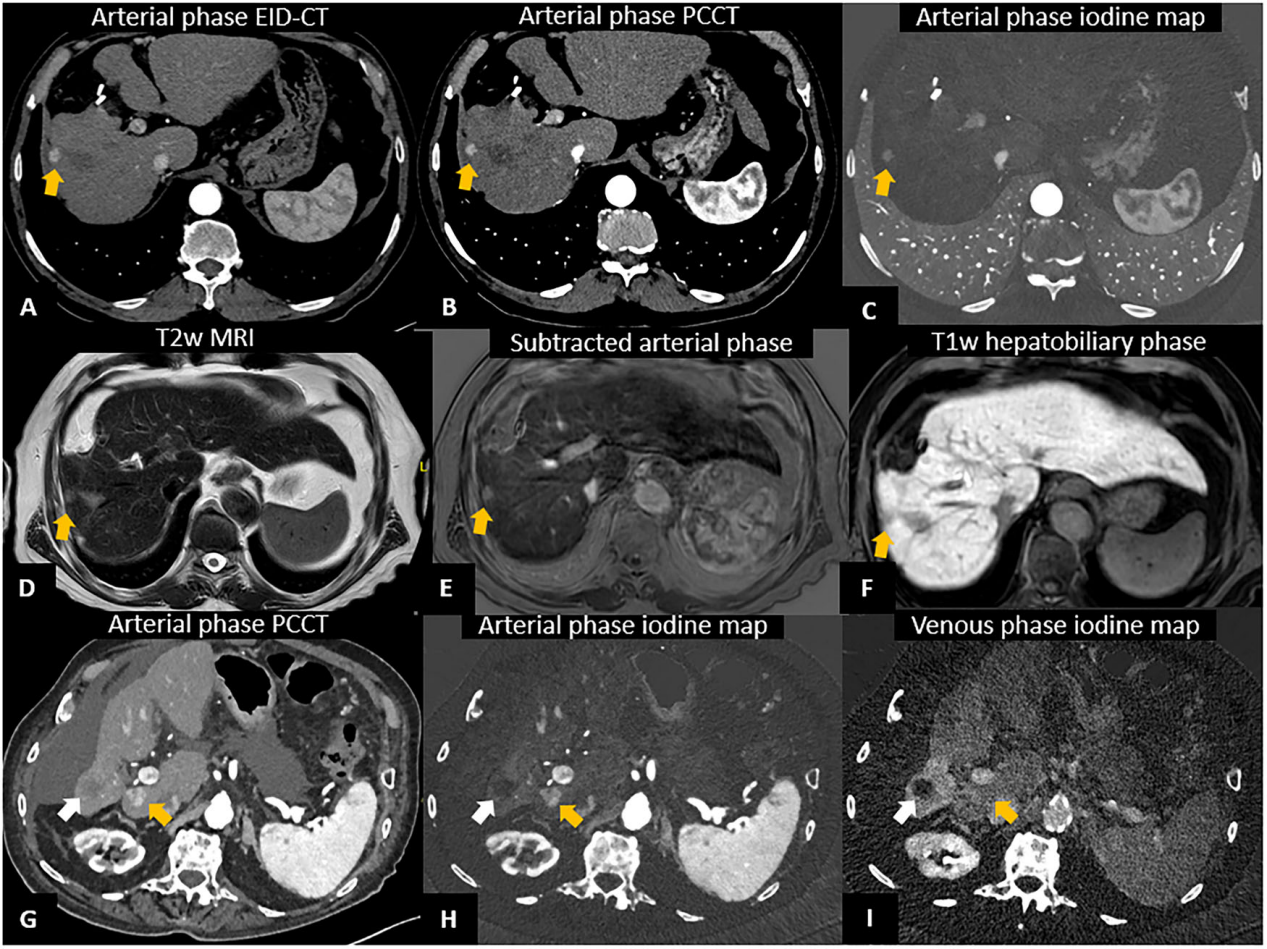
PCCT in hepatocellular carcinoma (HCC). A 75-year-old man with a previous resection for HCC and suspicion of disease recurrence. Both EID-CT (A) and PCCT (B) axial scans show a hypervascular nodule at the VI liver segment (yellow arrow in A and B), with a better margin definition at PCCT and increased iodine enhancement, further improved by iodine maps (C) that provided a subtraction-like image obtained from dynamic contrast enhancement MRI (F). PCCT findings were confirmed at MRI (D–F). A 94-year-old man with a history of HCC undergoing PCCT (G–I). Arterial (H) and venous (I) iodine maps show a hypodense round-shaped formation in the right hepatic lobe (white arrow in G–I), measuring 3 cm, consistent with the sequelae of HCC treated with stereotactic radiotherapy, with no enhancement suggestive for disease recurrence, but with an hypervascular nodule of VII segment (yellow arrow in G and H) with venous washout (yellow arrow in I) suggestive for HCC
